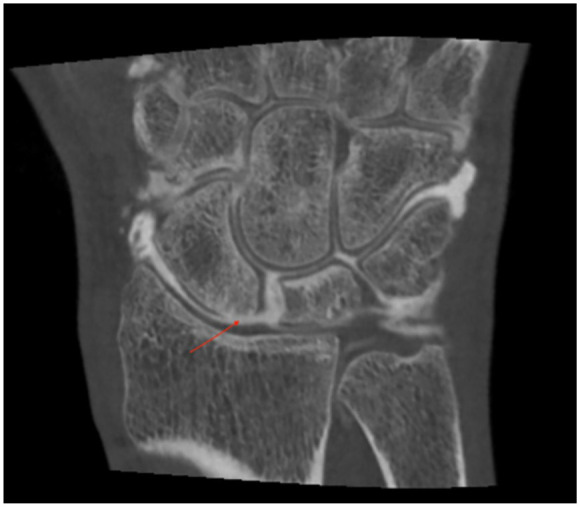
The use of intraarticular contrast fluid and cone-beam computer
